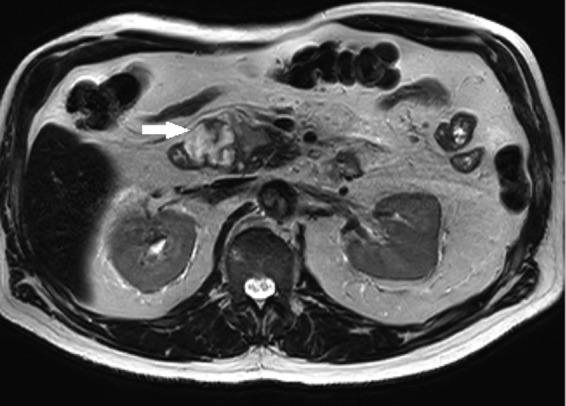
T2-weighted axial MRI demonstrates a well-defined mass (arrow) with high T2 signal components that has borders approximating the second portion of the duodenum and the uncinate process.
